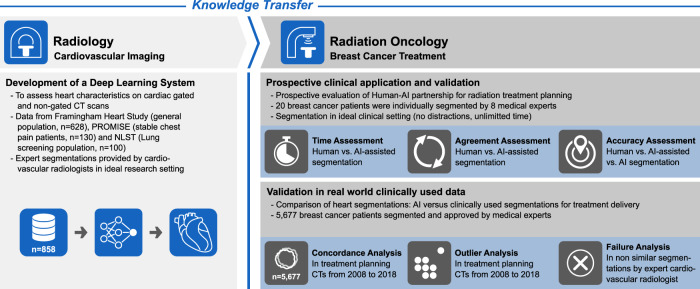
Study overview. A 3D deep-learning system was developed in cardiovascular radiology using CT scans from distinct and well-established cohorts. For training, medical experts segmented the heart in cardiac gated and non-gated CT scans. This specialized knowledge embedded in the deep-learning system was then transferred to radiation oncology and used to support treatment planning in patients with breast cancer.
